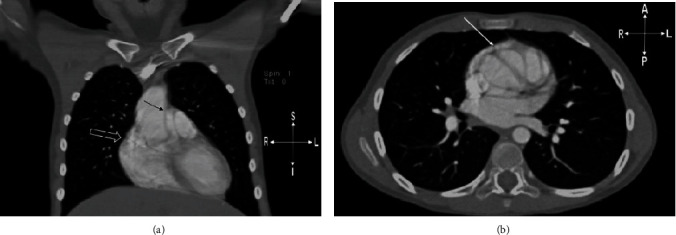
Repeat chest CT, coronal (a) and axial (b) views demonstrate a dilated ascending aorta (21.8 mm; Boston *Z*-score 2.84) and a suspected intimal dissection flap extending anteriorly off of the anterior cusp (thin arrow) just above the sinotubular junction. For comparison, the normal anatomic location for the RAA is highlighted (open arrow).
